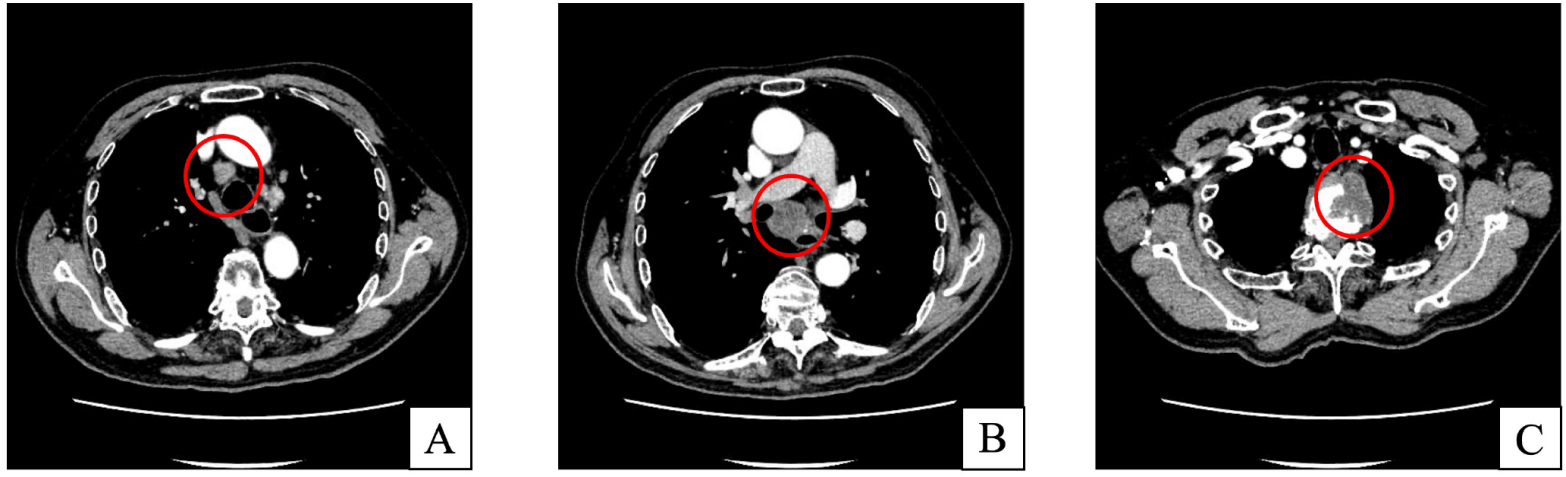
Contrast-enhanced chest CT of the mediastinum. Images obtained on August 29, 2024, show enlarged mediastinal lymph nodes at stations 4R (A) and 7 (B). A mass-like paravertebral soft-tissue lesion in the superior mediastinum shows well-circumscribed margins with peripheral rim enhancement and destructive changes involving adjacent vertebral bodies (C).
